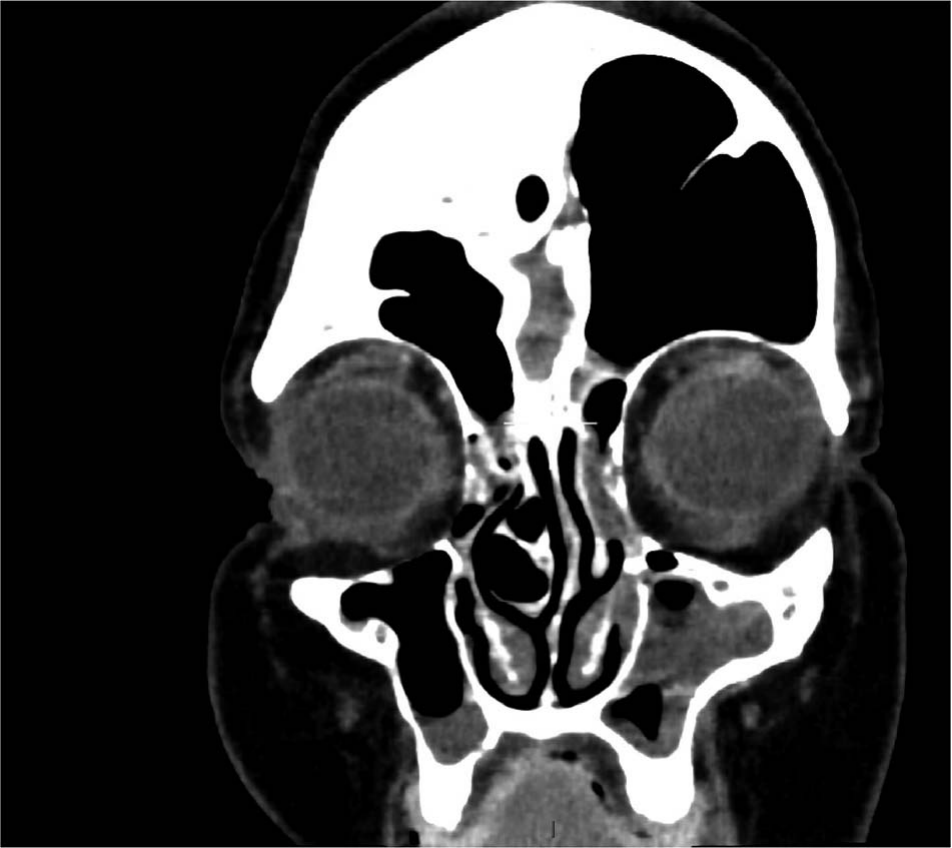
Coronal CT showing volume loss in the left hemisphere and enlarged sinus.
